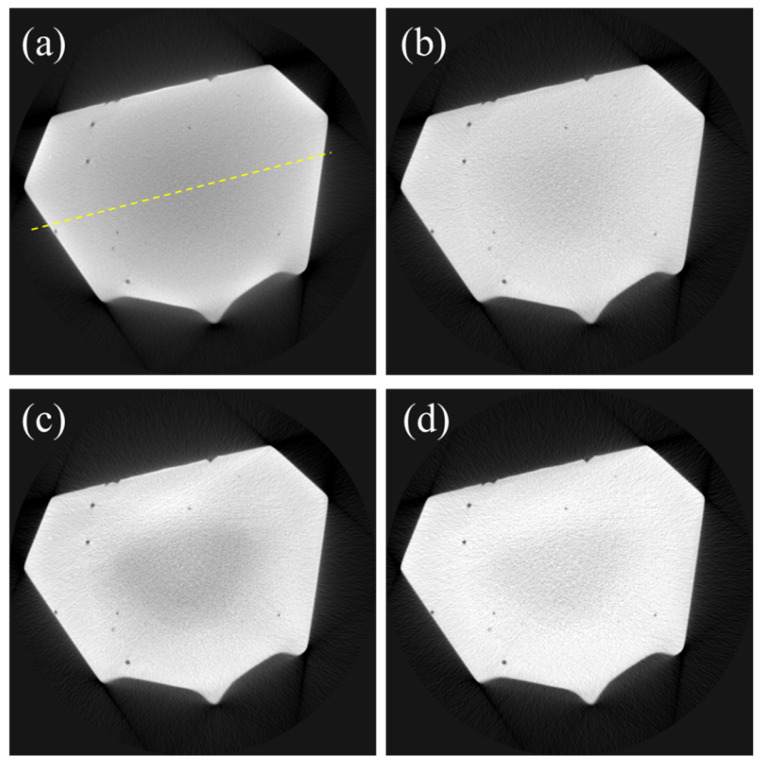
CT images of the base of the dental cast. (a) Uncorrected CT image, (b) DSC-based CT image, (c) SAC-based CT image, and (d) DSC-SAC-based CT image.
